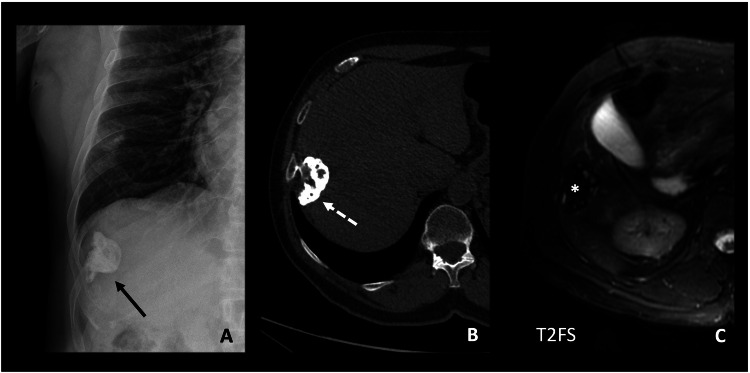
A 70-year-old man with a history of HME underwent abdominal ultrasound for elevated transaminases and a liver mass with very poorly defined contours was identified (not shown). Plain radiography (A), non-contrast CT (B), and MRI (C T2 with fat suppression) were performed. An osteochondroma dependent on the right 9th rib is identified, with calcification of the cartilaginous cap (continuous and dashed arrows on CT). The CT scan shows cortical and medullary continuity with the rib. The MRI shows hyperintense foci in T2, corresponding to the non-mineralised cartilaginous cap, and hypointense foci in T2, which correspond with the calcified cap visible on the CT (*). Since the patient was asymptomatic, a conservative approach was decided
